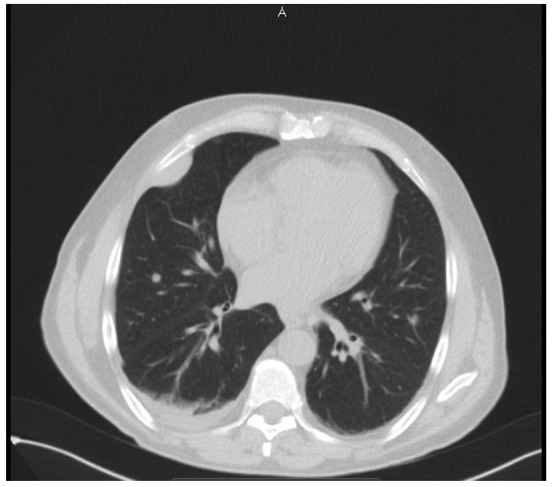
Computed tomography image of the thorax revealing the presence of multiple pleuropulmonary metastatic nodules and pleural effusion.
